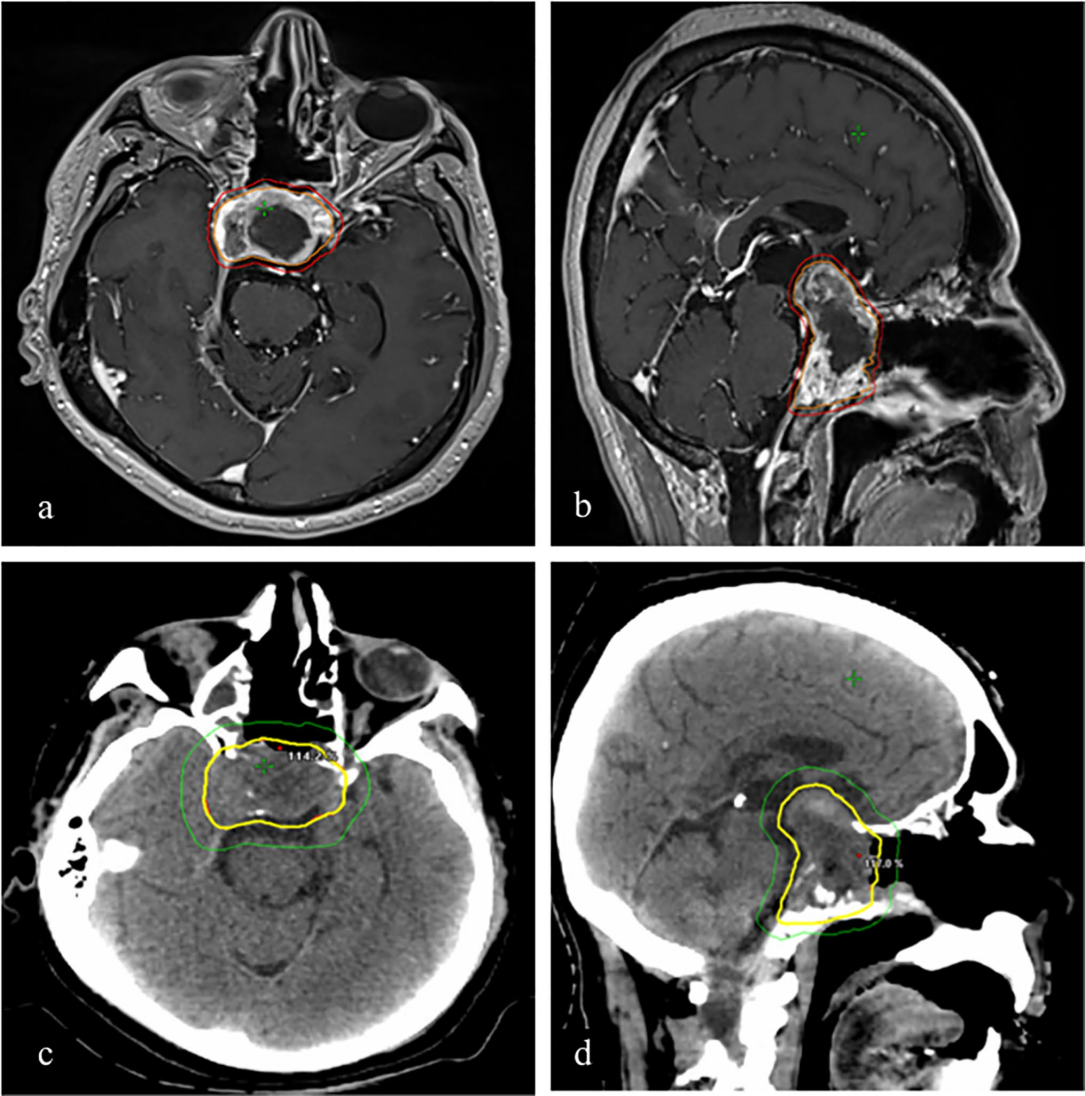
a & b Planning Target Volume (PTV) consisted of resection cavity and residual tumor identified on T1 post contrast sequence of post op MRI brain (outlined in orange) plus 2 mm margin (outlined in red). A dose of 2500 cGy in 5 fractions was prescribed to PTV by Stereotactic Radiation Therapy using 4-arc VMAT with 6MV Flatting Filter Free (FFF) photon beams to cover the PTV volume with 100% (2500 cGy) isodose line (in yellow) on planning CT head (c & d)
